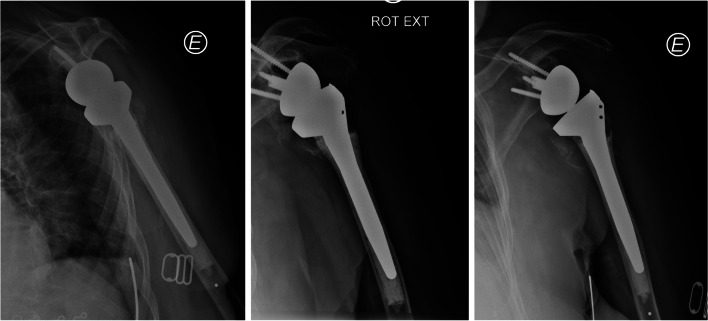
X-ray evaluation of the conventional humeral stem (Aequalis Reversed II implant; Tornier, Memphis, TN, USA)
